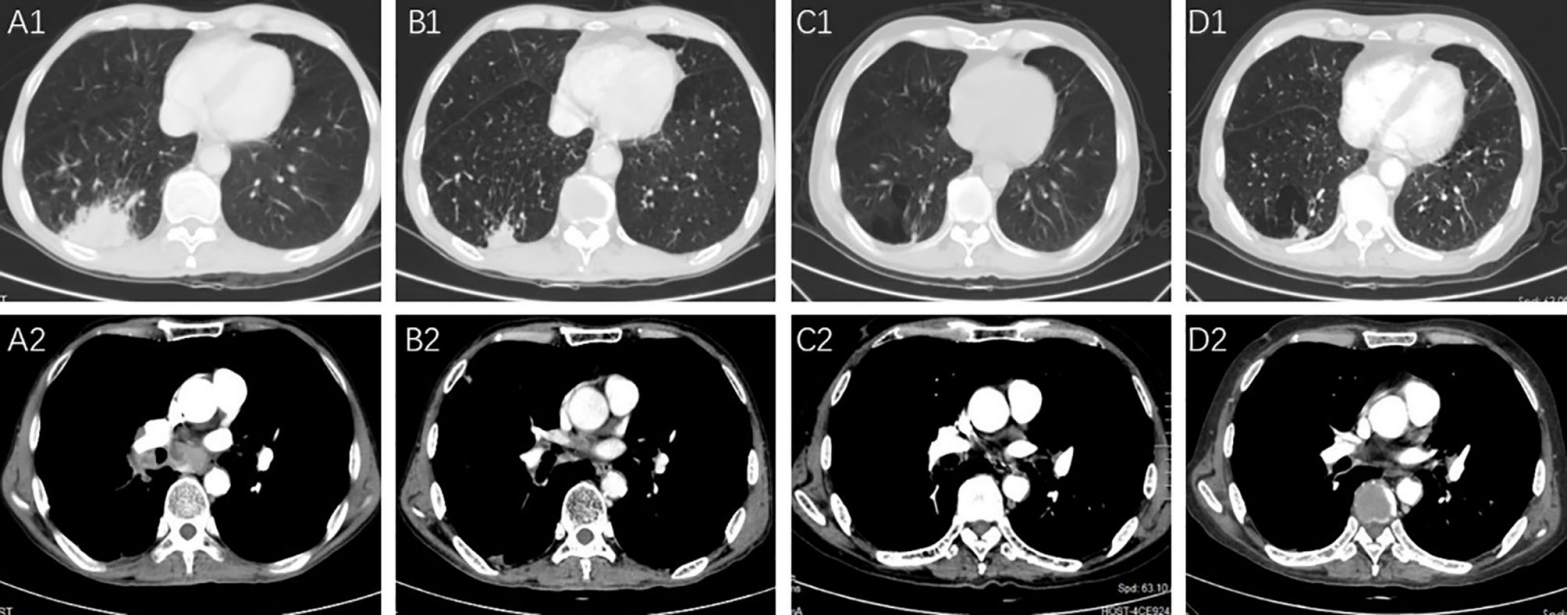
The pulmonary lesions and lymph node metastases before and after the PD-L1 treatment. (A1, A2) Right lower pulmonary lesions and swollen lymph nodes were revealed in the CT images before starting the chemotherapy in May 2018. (B1, B2) Partial response of the lesions in the right lower lobe and swollen lymph nodes was shown after 6 cycles of chemotherapy and atezolizumab treatment in August 2018; (C1, C2) the lesions showed continuous partial response after 36 cycles of pemetrexed and atezolizumab; (D1, D2) the lesions indicated a decreased size in March 2021.
